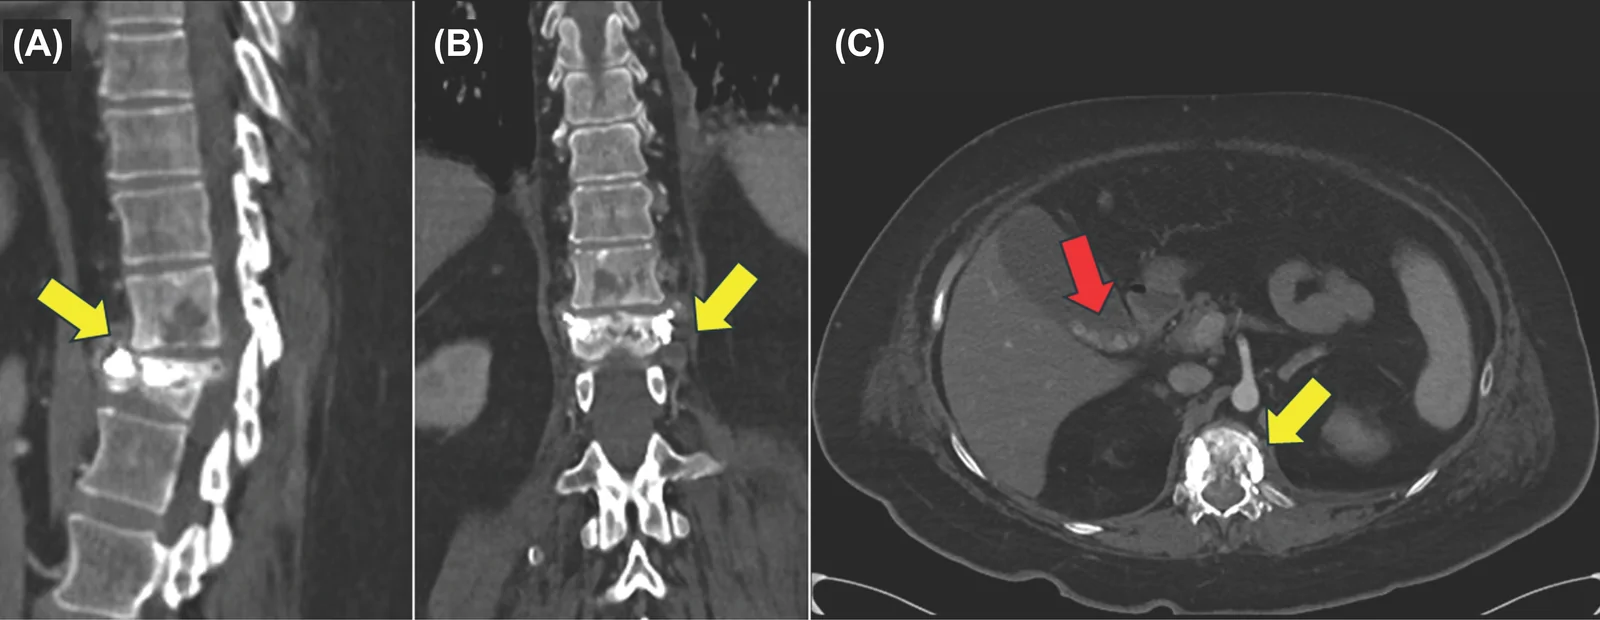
Abdominopelvic CT sagittal (A), coronal (B), and axial images (C). T12 insufficiency fracture with cement *in situ* (yellow arrow). Multiple hyperdense gallstones compatible with cholelithiasis (red arrow). Abbreviation: CT, computed tomography.
